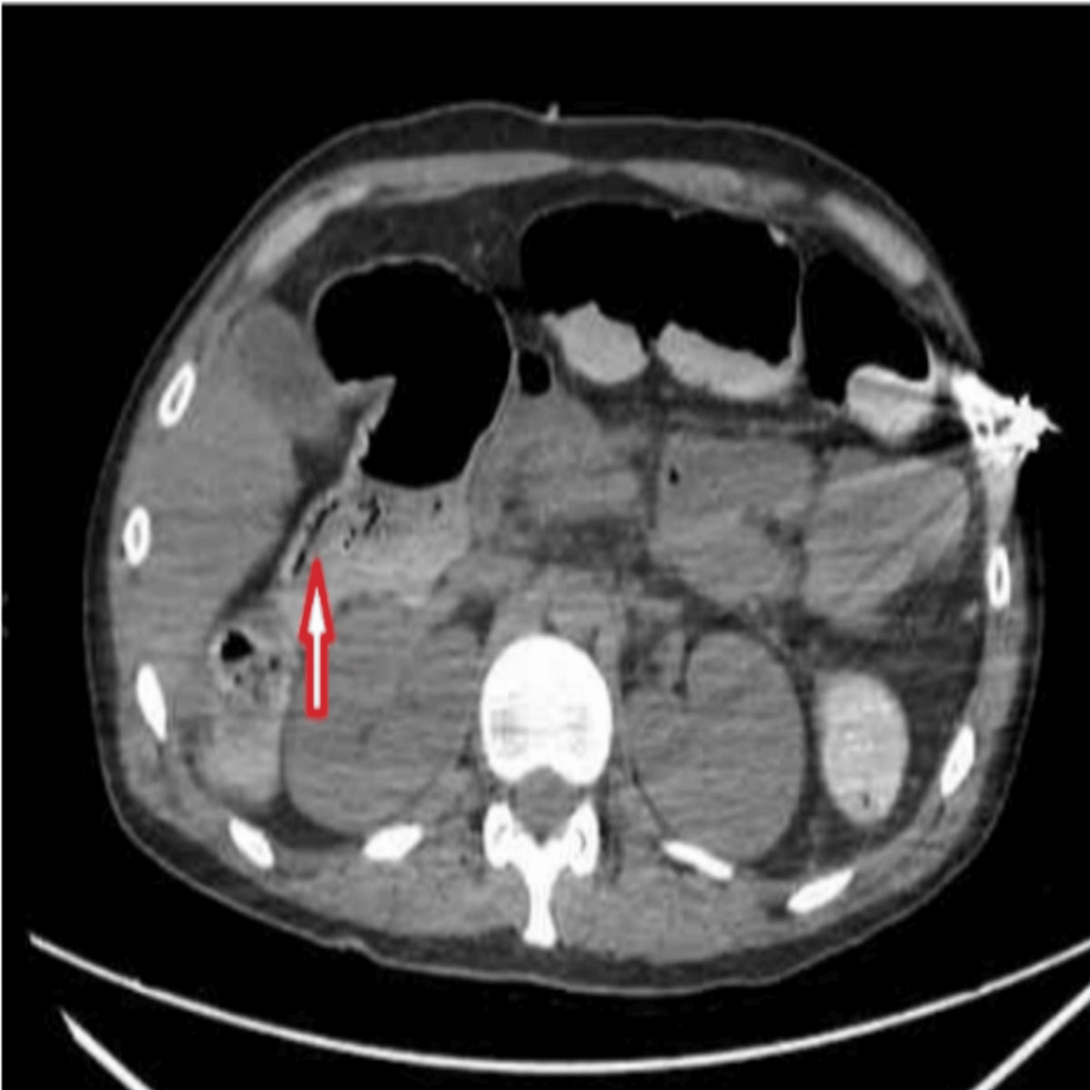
CT abdomen and pelvis, axial (transverse) section, showing pneumatosis intestinalis (arrow).
